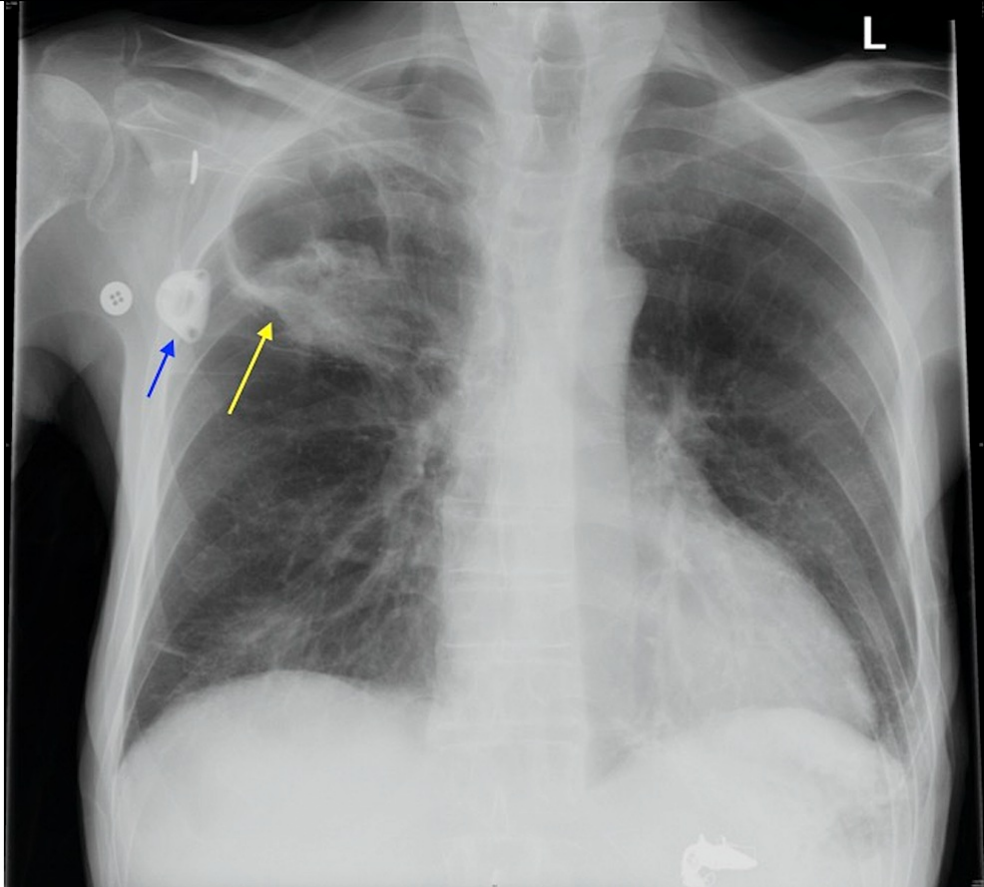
Chest radiograph demonstrating a right-sided apical cavitary lesion (yellow arrow) and a chemotherapy port (blue arrow).
